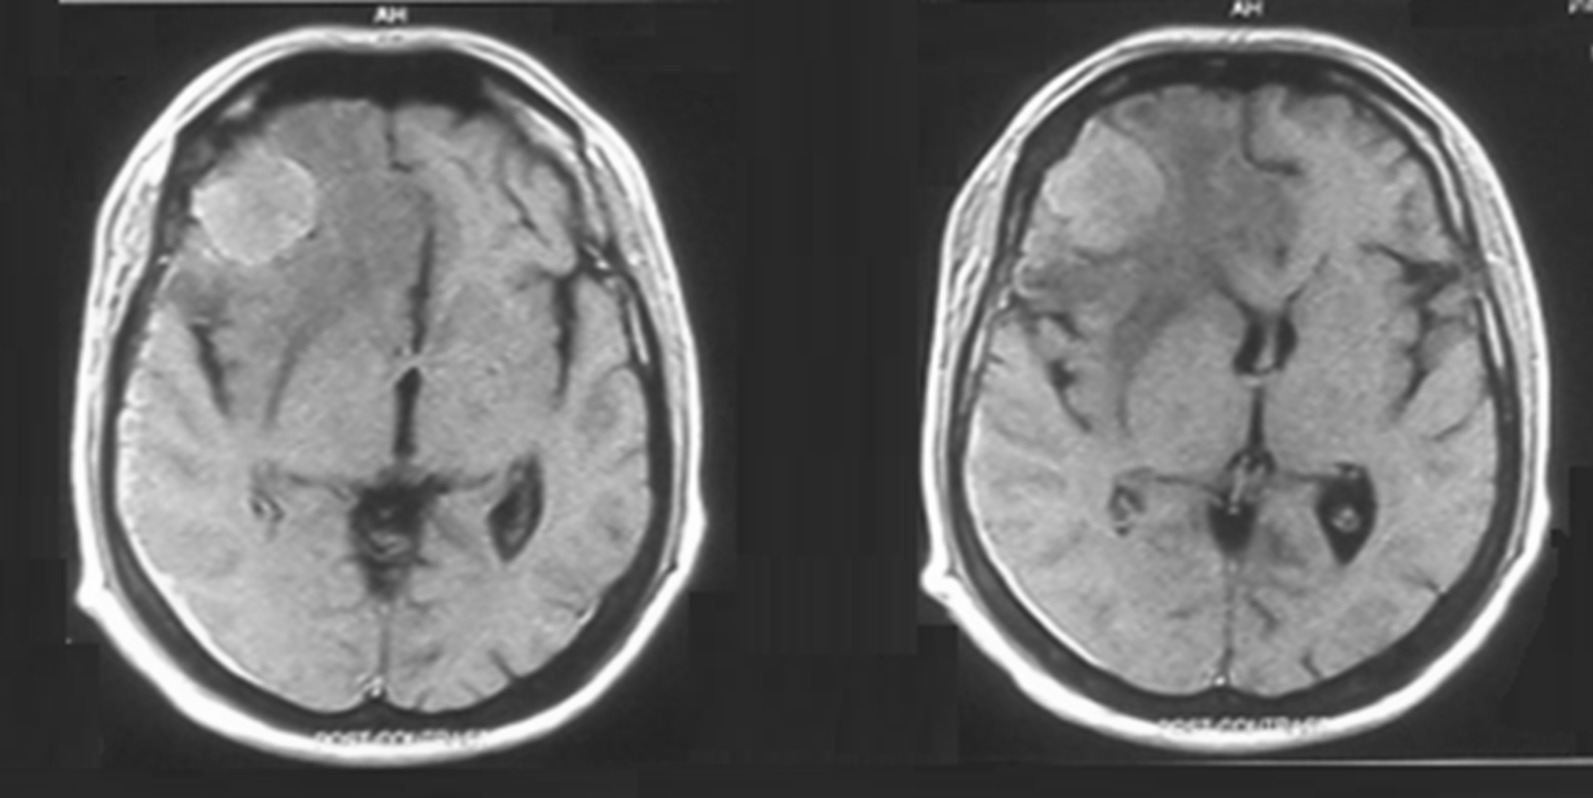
Radiographical presentation showing space-occupying lesion in right parietal region
